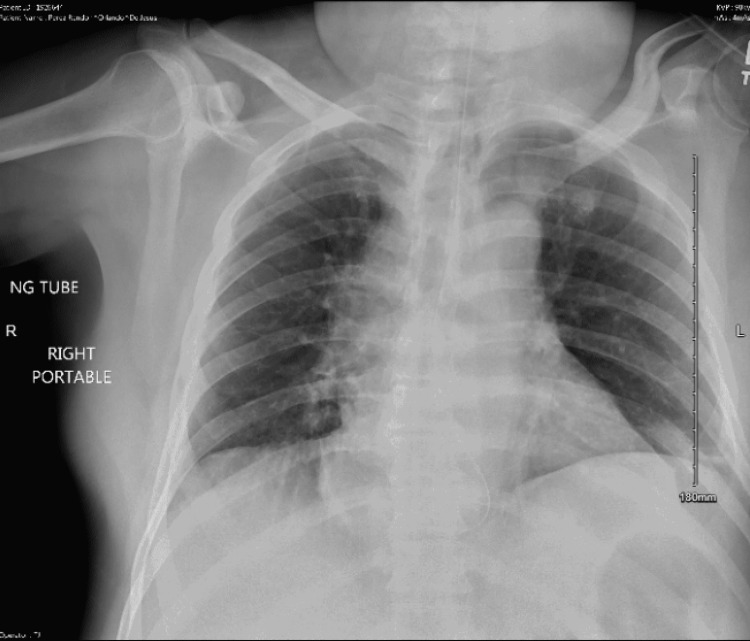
Chest X-ray on postoperative day one
